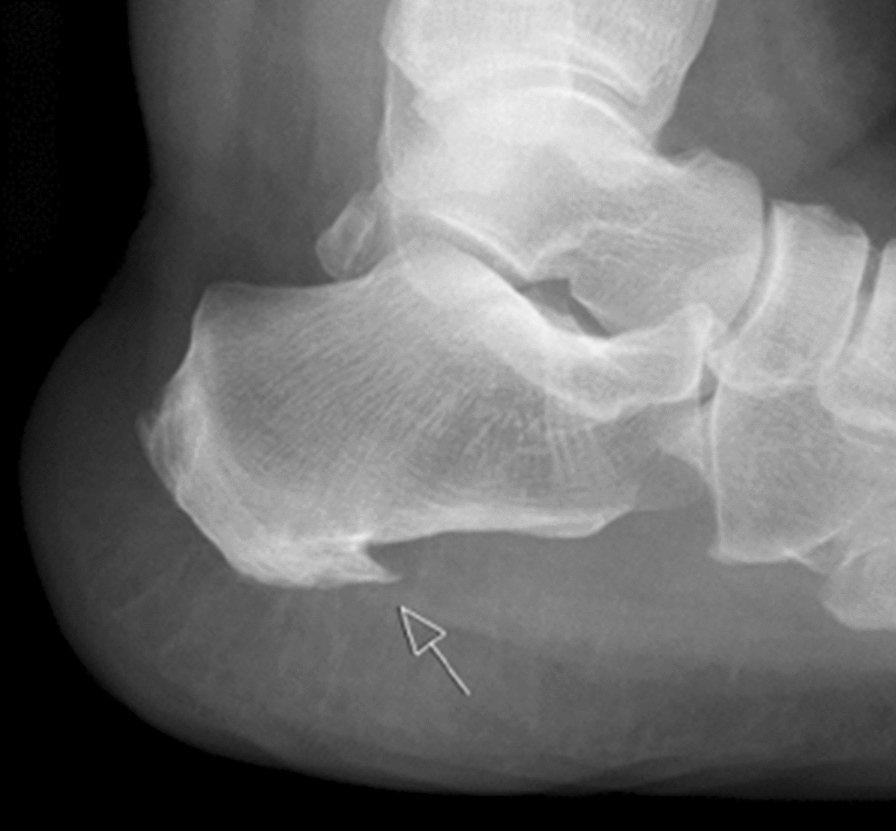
X-ray of plantar enthesophyte. The X-ray of the calcaneus shows a heel spur with an inflammatory reaction surrounding the insertion of the inferior aponeurosis (arrow)
